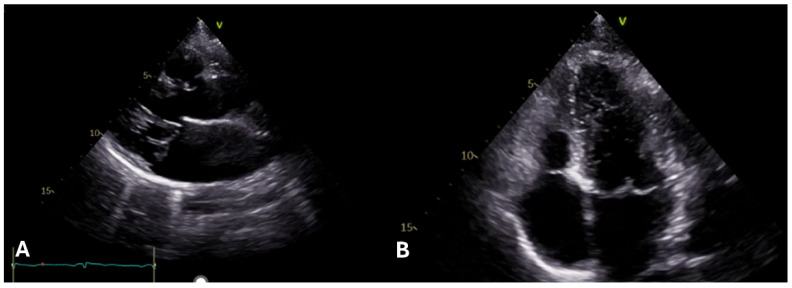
(A,B) 2D transthoracic echocardiography (TTE) images of a young adult with Marfan syndrome. Image (A) displays mitral valve prolapse in the long parasternal axis view, while image (B) shows the mitral valve prolapse in the four-chamber view.
